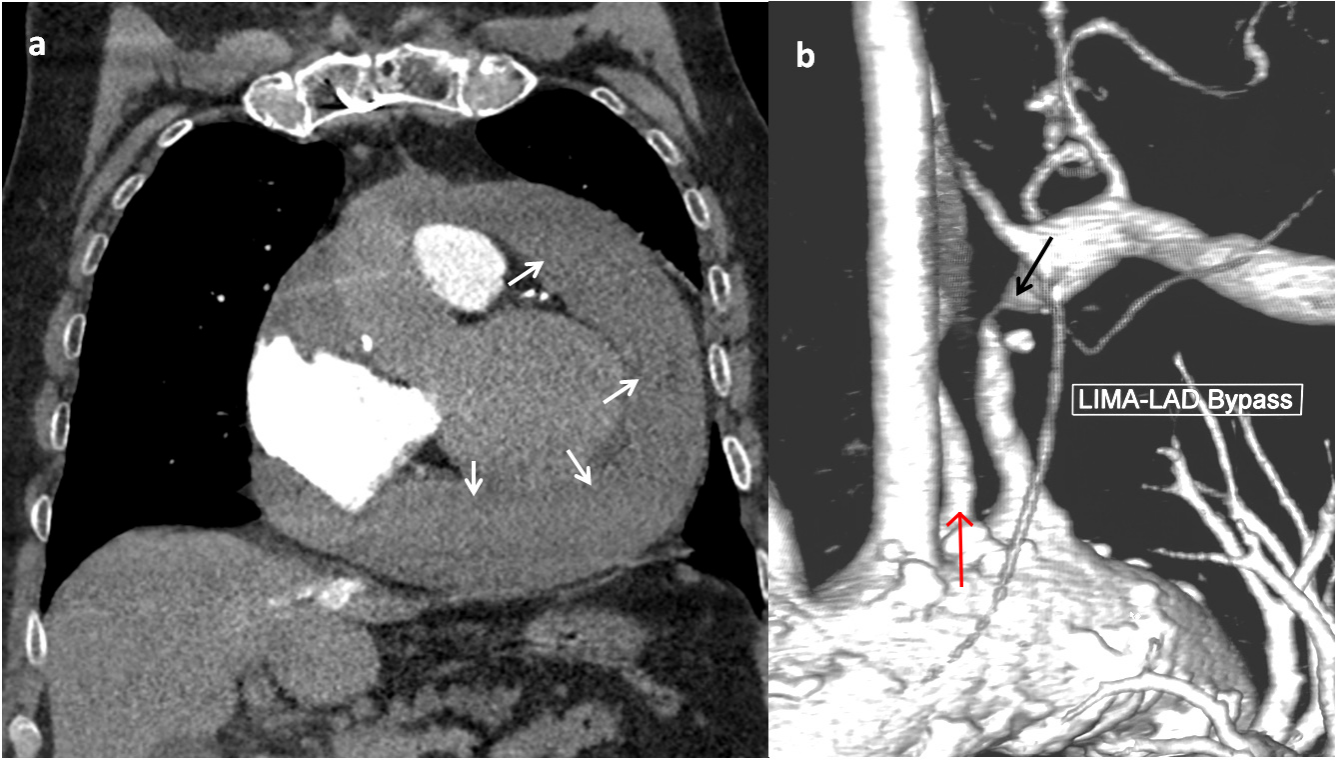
(A) Contrast-enhanced chest computed tomography (CT) image, multiplanar reconstruction, coronal plane, extensive pericardial effusion (white arrows). (B) CT angiography, 3D Volume Render image, high-grade stenosis of the left subclavian artery next to the left internal mammary artery (LIMA) bypass graft (black arrow), anomalous origin of the left vertebral artery from the aorta (red arrow).
